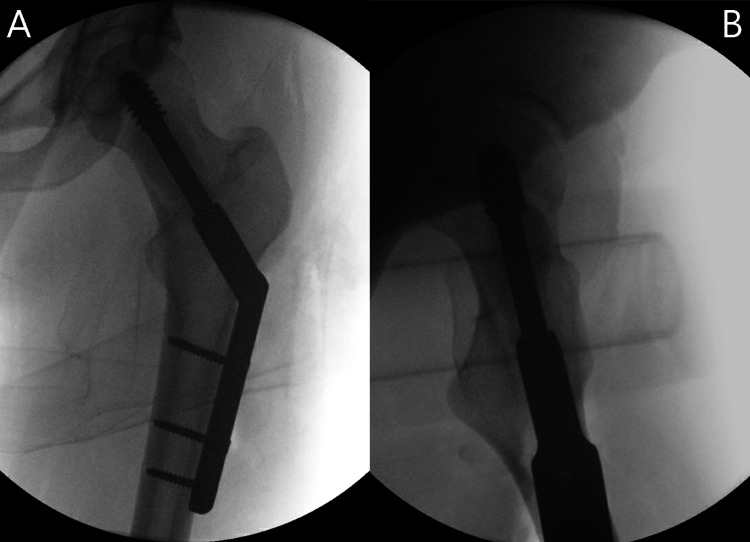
Radiographic anteroposterior (A) and lateral (B) views of the left hip intraoperatively after curettage with use of PRO-DENSE™ and operative fixation
